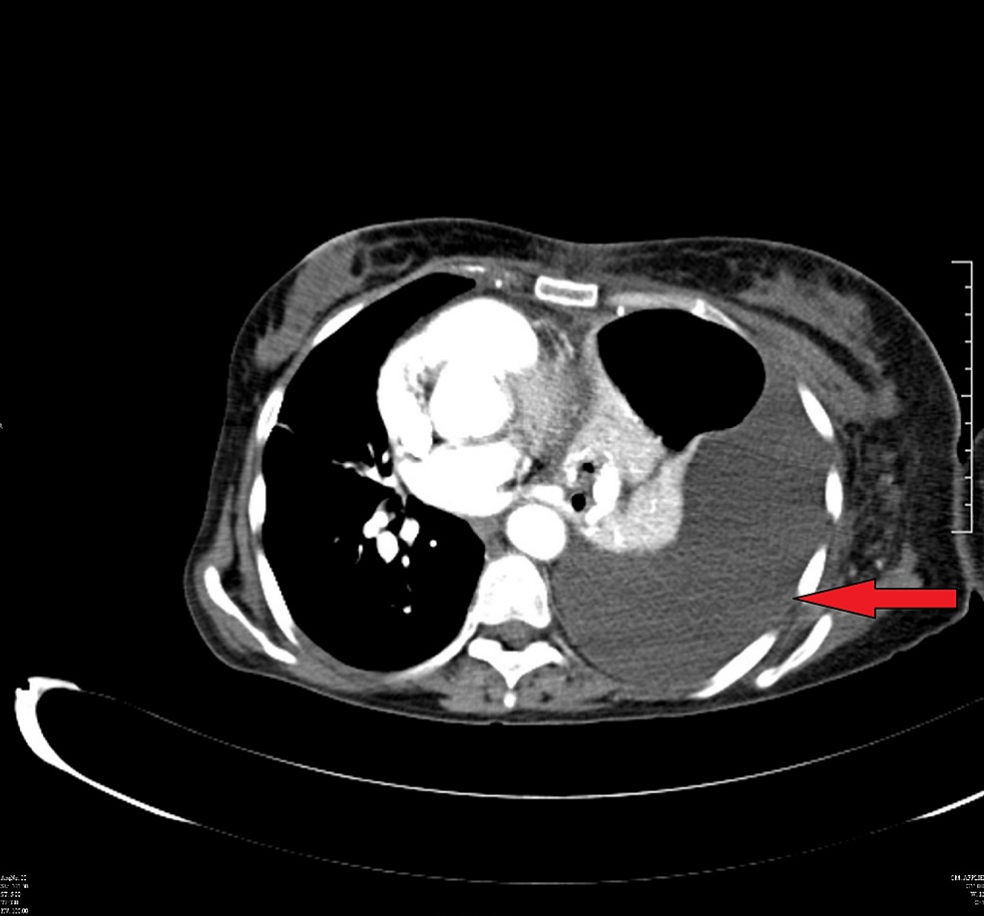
CT chest showing the left-sided pleural effusion.
